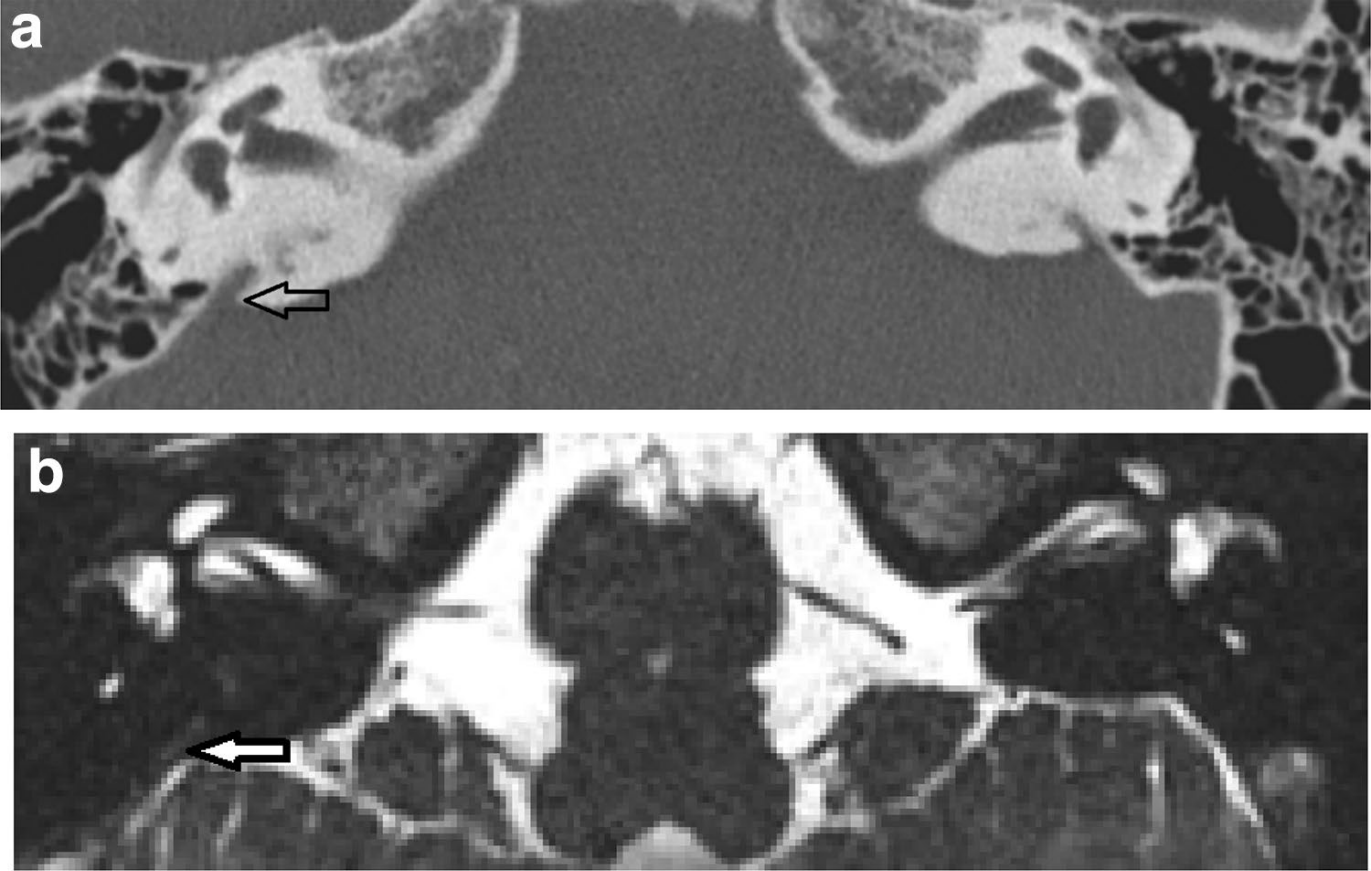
CT positive for LVAS but MRI negative for LESA. Axial CT (a) and T2 CISS axial MR image (b) demonstrates a case in which there was 2 mm measurement at the operculum on CT, thus Cincinnati criteria positive for LVAS (black open arrow) but not on MRI (white filled arrow)
